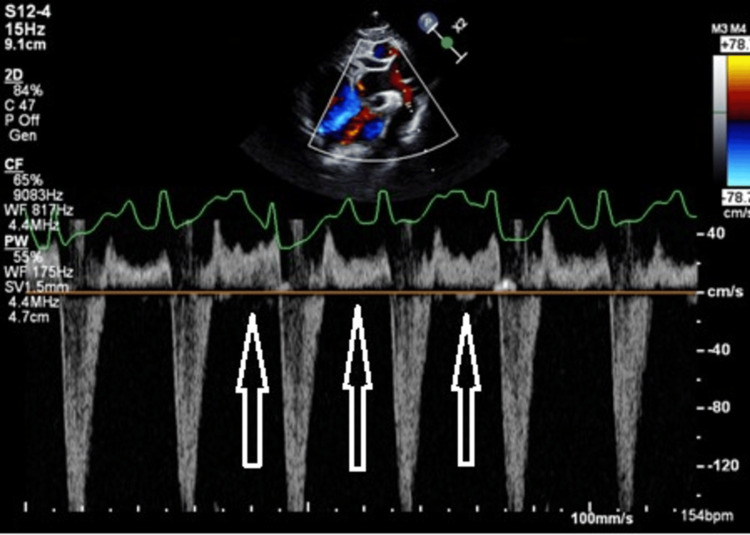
Pulse wave Doppler in the transverse aortic arch. There is unexplained diastolic flow reversal in the absence of a PDA which raised suspicion of VOGM. Diastole and systole are labeled (arrows). PDA: patent ductus arteriosus; VGAM: vein of Galen aneurysmal malformation
